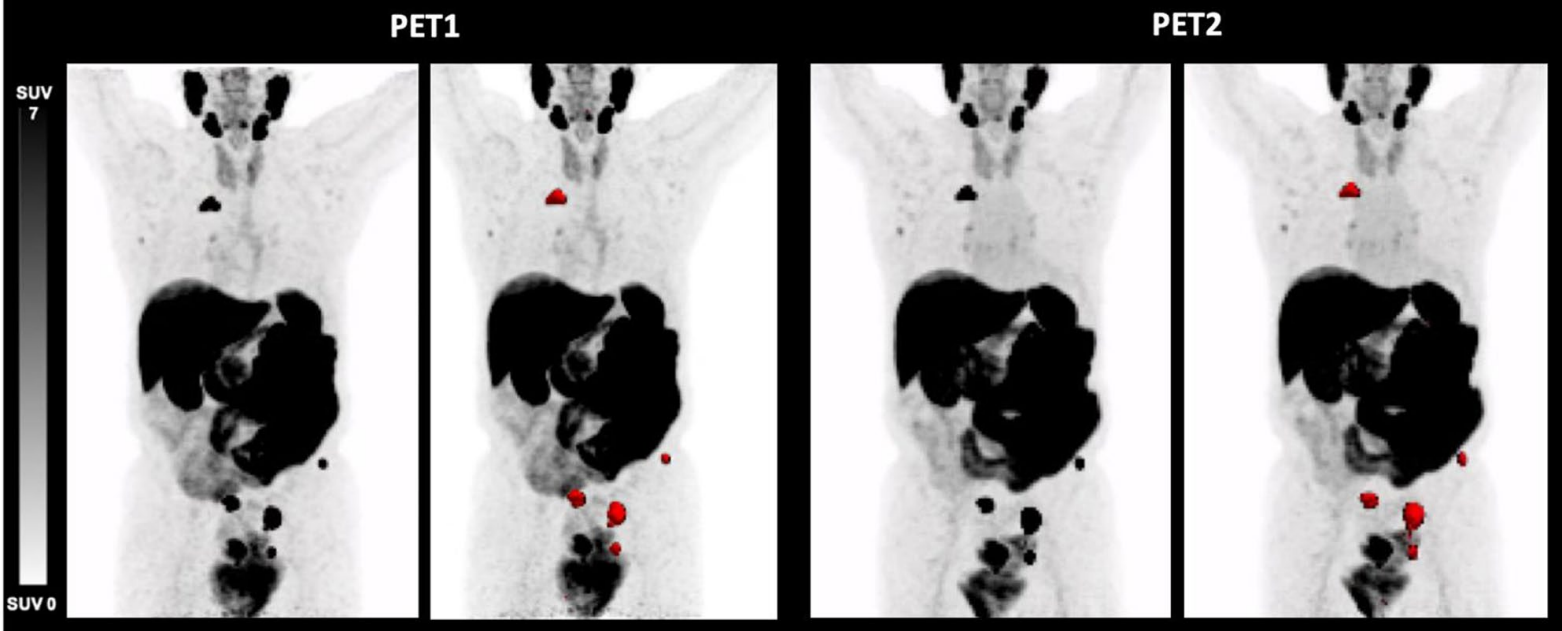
[^18^F]PSMA-1007 PET/CT derived maximum intensity projection (MIP) of a 85-year-old patient with metastatic prostate cancer before (PET1) and after (PET2) initiation of apalutamide therapy (time difference 2.70 months). TTV (+ 16%), SUV_max_ (+ 172%) and SUV_mean_ (+ 113%) increased, PSA level decreased from 18.70 ng/mL to 8.00 ng/mL. This patient was rated as SD according to RECIP 1.0
